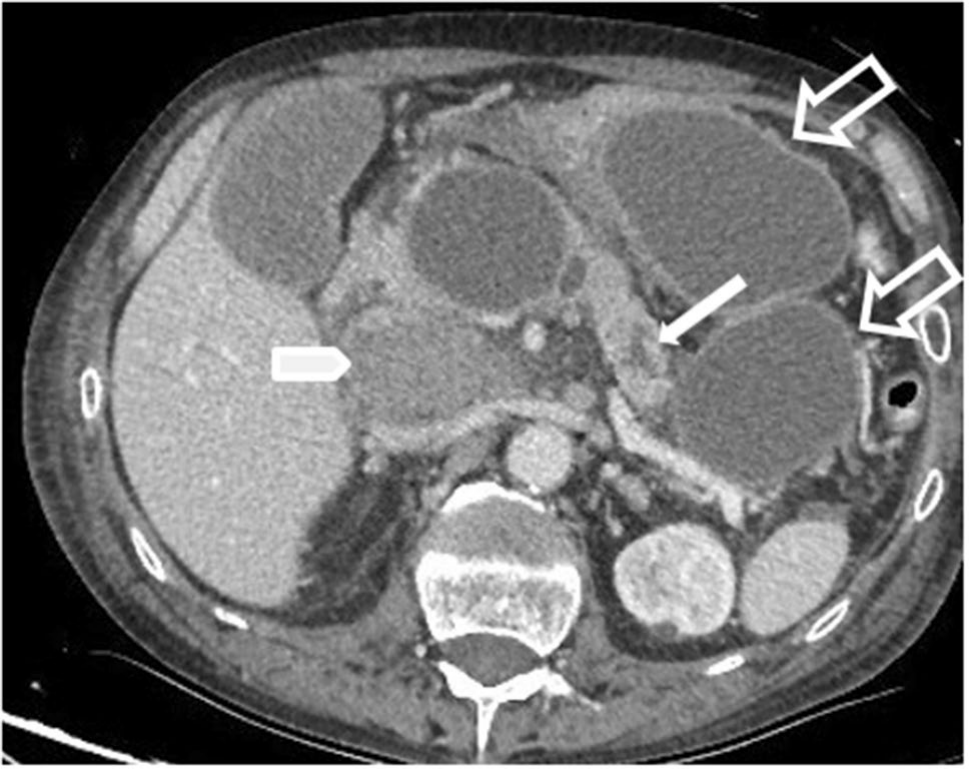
Pseudocyst: 61 years old man, with history of alcohol abuse and hospitalized for necrotizing pancreatitis. Contrast-enhanced CT scan after 4 weeks from the onset, show a hyperdense collection in the retroperitoneum, behind the head of the pancreas and the duodenum (arrow head) suggesting previous bleeding. No signs of active bleeding were evident. Other pseudocysts are localized in the peri-pancreatic fat, between body and tail (empty arrows). Wirsung duct is also dilated (thin arrow)
